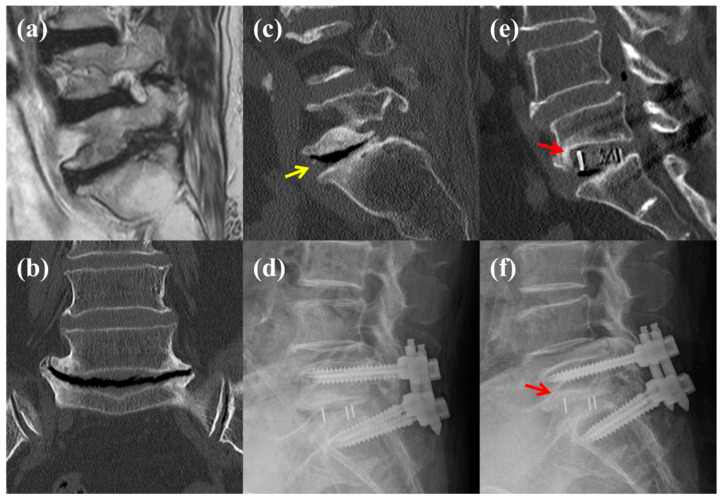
A representative case of subsidence following oblique lateral interbody fusion surgery in a patient with vacuum phenomenon (VP) grade 3 at the L5–S1 level. The patient presented with severe radiculopathy in the right L5 dermatome, causing claudication and radiating pain. Conservative treatment was unsuccessful, and surgery was performed. (a) Sagittal magnetic resonance imaging revealed right L5 nerve root compression owing to L5–S1 foraminal stenosis. (b) Coronal computed tomography (CT) view showed collapse across the entire L5–S1 disc, bilateral disc space spurring, and a prominent VP. (c) Sagittal CT view demonstrated disc collapse, endplate sclerosis, and VP grade 3 at the L5–S1 (yellow arrow). (d) Immediate postoperative plain lateral radiograph confirmed the disc space height restoration because of the cage. (e,f) At 2 months postoperatively, subsidence was observed (red arrow), with CT sagittal and plain lateral radiographs revealing L5 endplate damage and cage subsidence into the L5 vertebral body.
